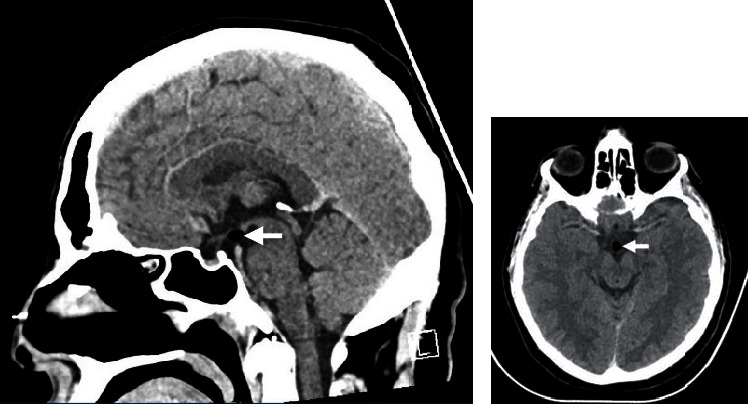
(a) Sagittal brain CT. (b) Axial brain CT. (a) and (b) The images demonstrate a small hypodense lesion, measuring 5 × 7 mm, consistent with hypothalamic lipoma.
